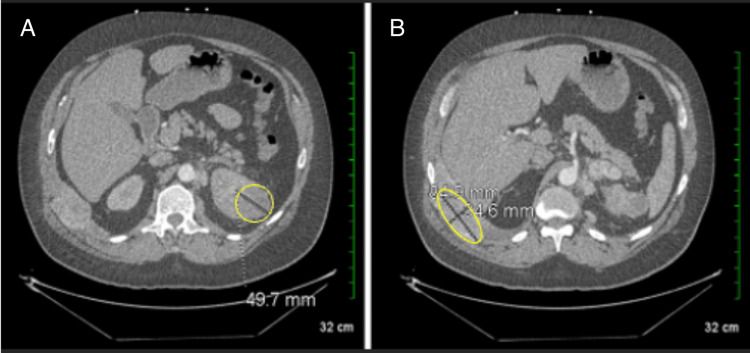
(A) CTA showing a left upper pole renal mass measuring 49.7 mm (yellow circle), with stable surrounding mass-like densities indicative of adenopathy. (B) Imaging of the right chest wall revealing a mass between the 10th and 11th ribs, measuring 84.9×34.6 mm (yellow circle), accompanied by destructive changes in the right 11th rib and a fracture of the right 10th rib. CTA: computed tomography angiography
